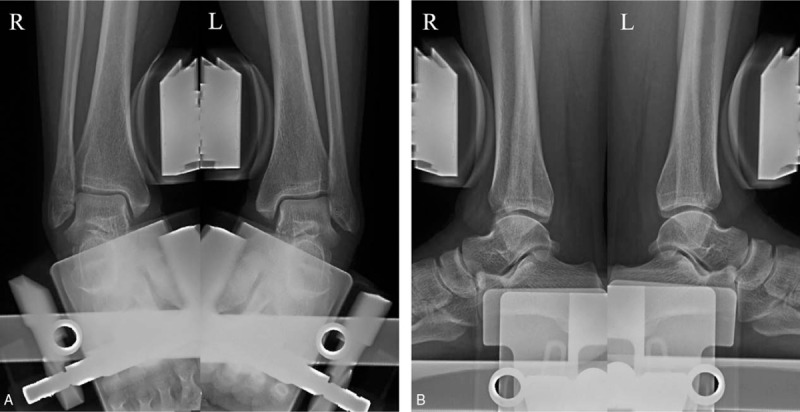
Preoperative anterior drawer test (A) and varus stress view (B) radiographs.
